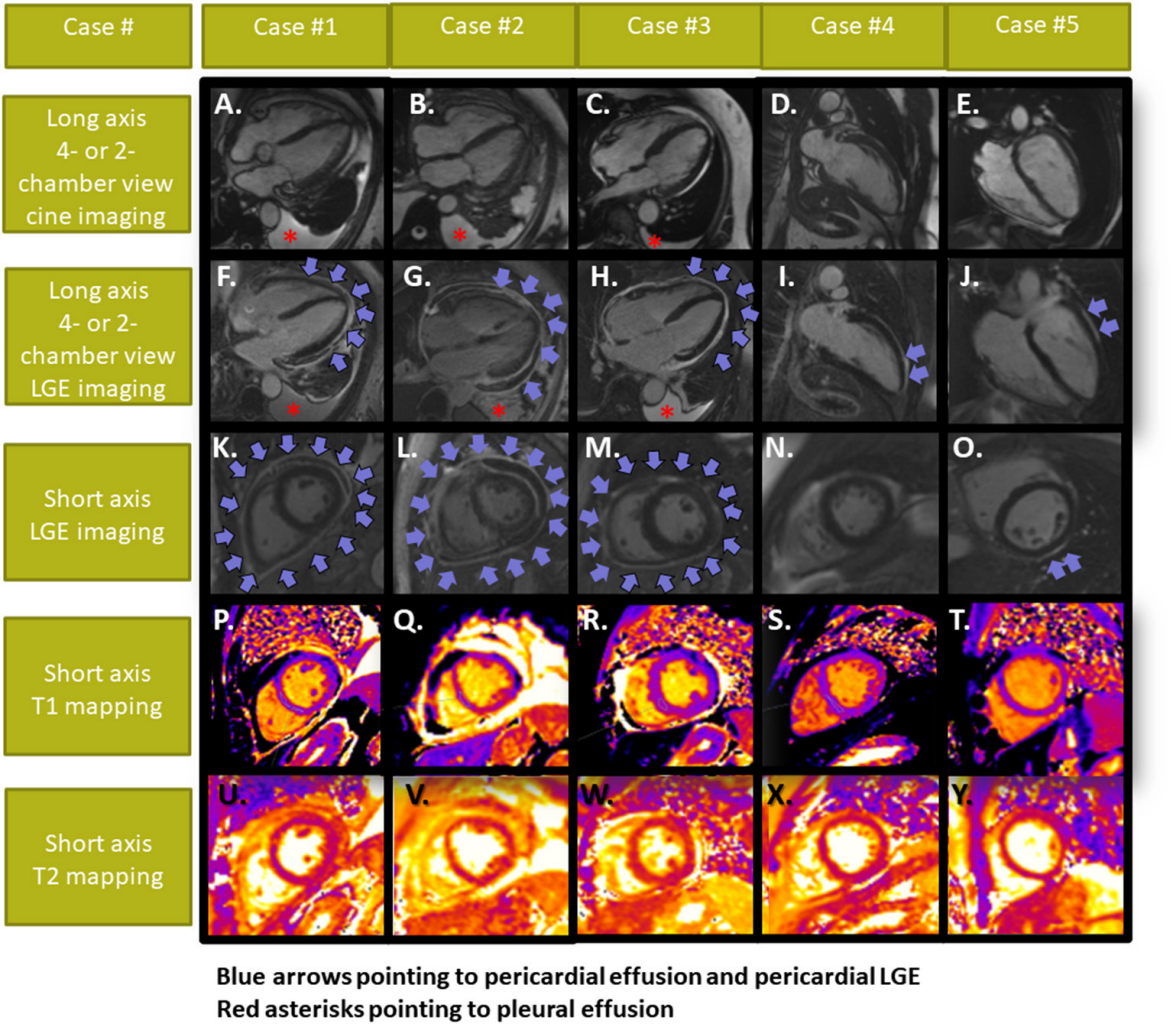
Cardiac MRI images of patients #1–5. Cine images are displayed in (A–E). All patients showed pericardial LGE, which was either diffuse [(F,K) in patient #1; (G,L) in patient #2 and (H,M) in patient #3] or focal [(I, N) in patient #4 and (J,O) in patient #5], whereas myocardial LGE or elevated T1- and T2-values (P–Y) were not present with any of our patients. Pericardial and pleura effusion was present in patients #1–3. Patient #2 developed signs of a pericardial tamponade and underwent urgent pericardiocentesis (arrows depicting pericardial effusion and LGE in cases #1–3 and pericardial LGE without effusion in cases #4,5; asterisks pointing to the pleura effusions in cases #1–3).
